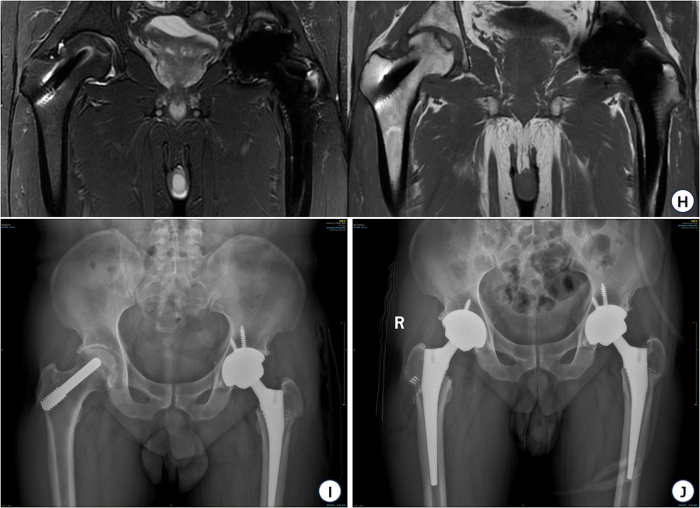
Postoperative imaging of the patient. (A) 2024-09, preoperative MRI of the right hip. (B) 2024-09, preoperative bilateral anteroposterior hip positions of the right hip. (C) 2024-09, postoperative bilateral anteroposterior hip positions of the right hip.
